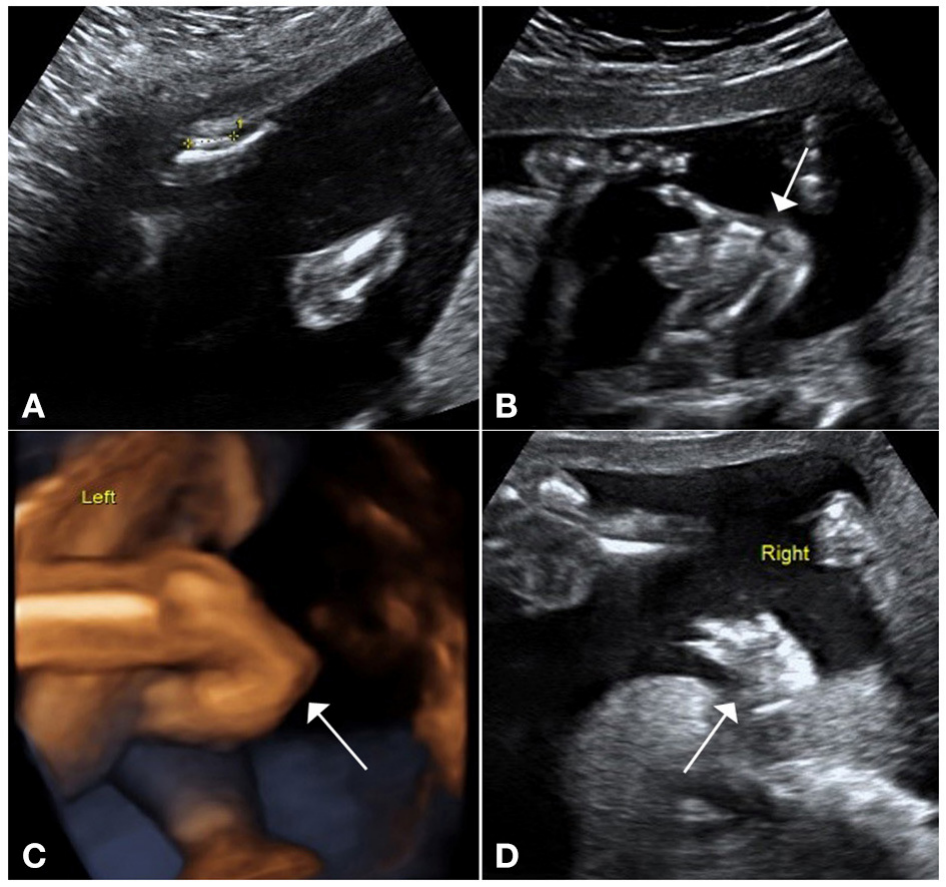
Ultrasound pictures demonstrate upper-limb malformations. (A) Ultrasound demonstrates shortness of the radius and ulna, especially the radius. (B) Ultrasound and (C) 3D views show that the hands had the inability to supinate. (D) Right thumb was missing in ultrasound imaging.
